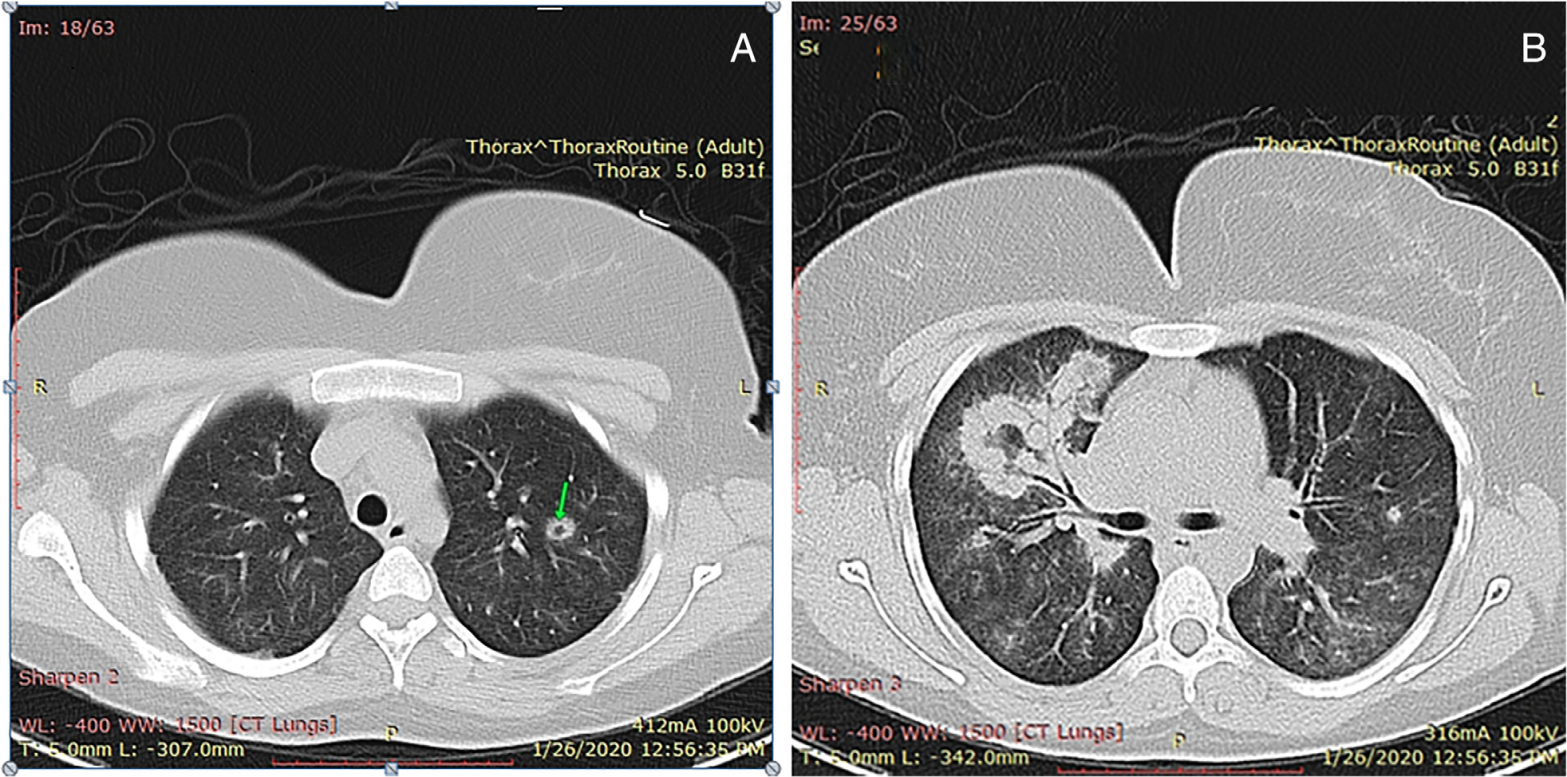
(A) Axial image of chest computed tomography (CT) scan showed a diffusely distributed pattern of centrilobular perivascular ground‐glass opacities and multiple nodular densities, in which one of them shows central cavitation (arrow). (B) CT scan revealed a segmental consolidation in the anterior segment of the right lung and irregular necrosis with multiple nodules and ground‐glass opacities in both lungs.
